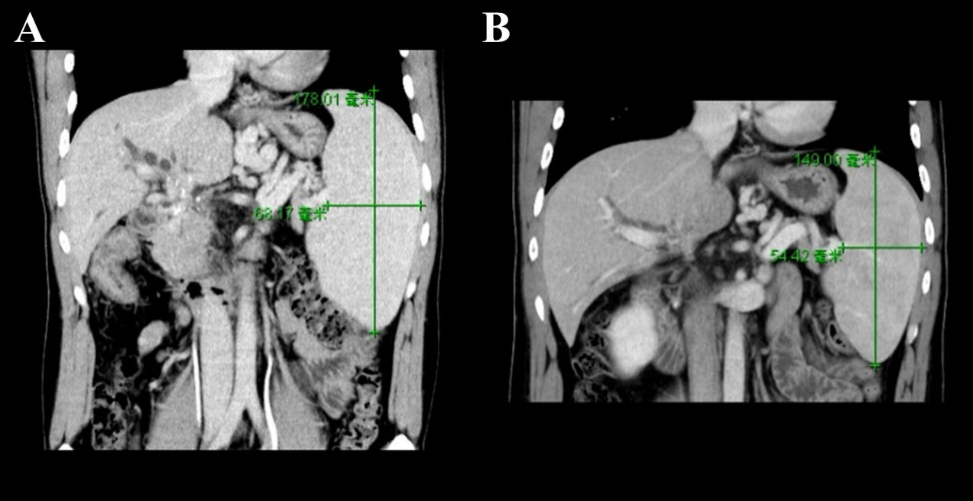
At the 1-year postoperative follow-up, enhanced CT scans of 6 patients showed that the long axis of the spleen was reduced to ≥ 2 cm. The comparison of splenic volume between a preoperative (A) and postoperative (B) MESO-REX patient showed that the splenic volume decreased significantly after the operation suggesting that the MESO-REX intervention was effective
